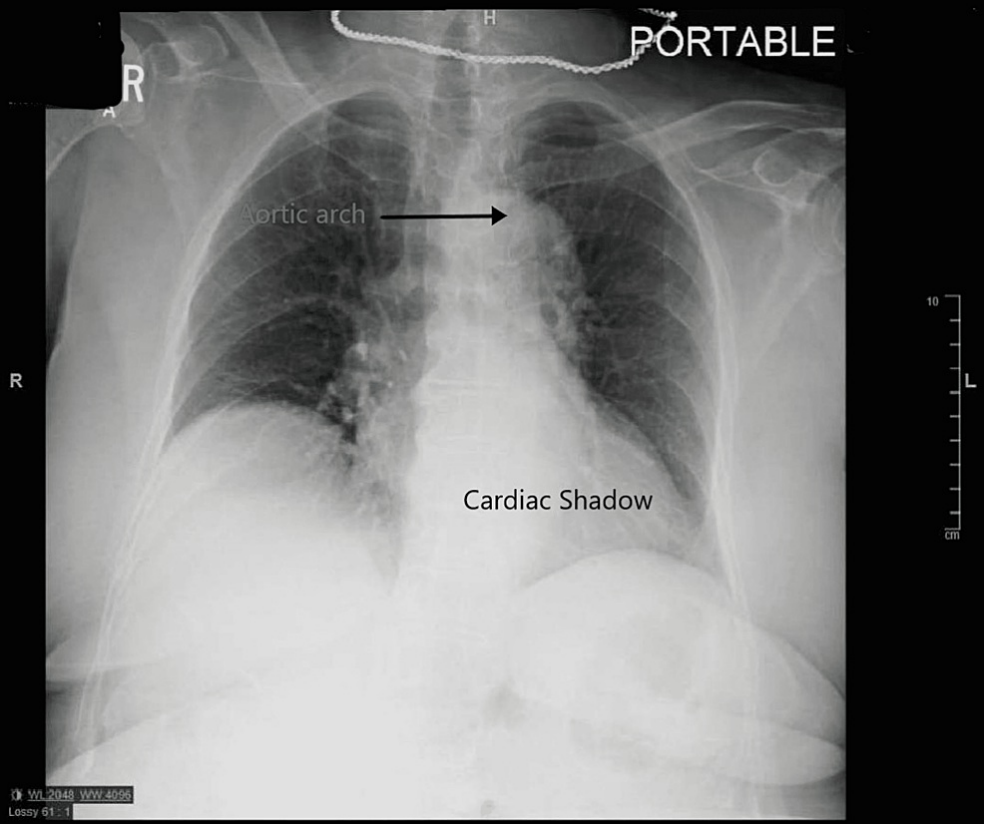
Chest radiograph showed a borderline enlarged heart with the tortuous and calcified aorta.
